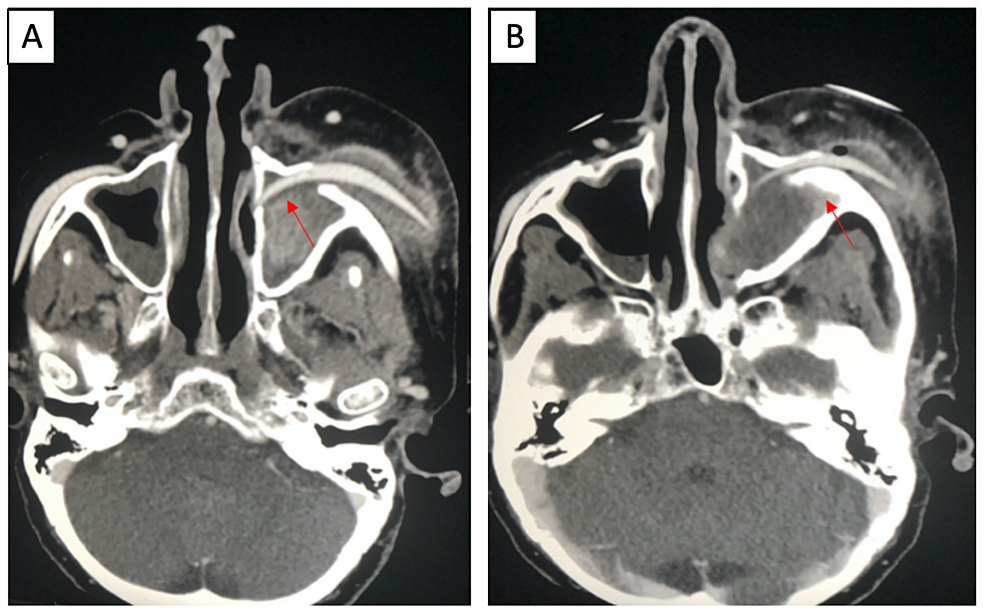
Enhanced CT, axial view. Enhanced CT displaying left-sided facial swelling, edema, and abscess. Penetration of the left malar implant into the left maxillary sinus and abut the lamina papyracea is noted (A) with left zygomatic bony erosion secondary to osteomyelitis (B).
